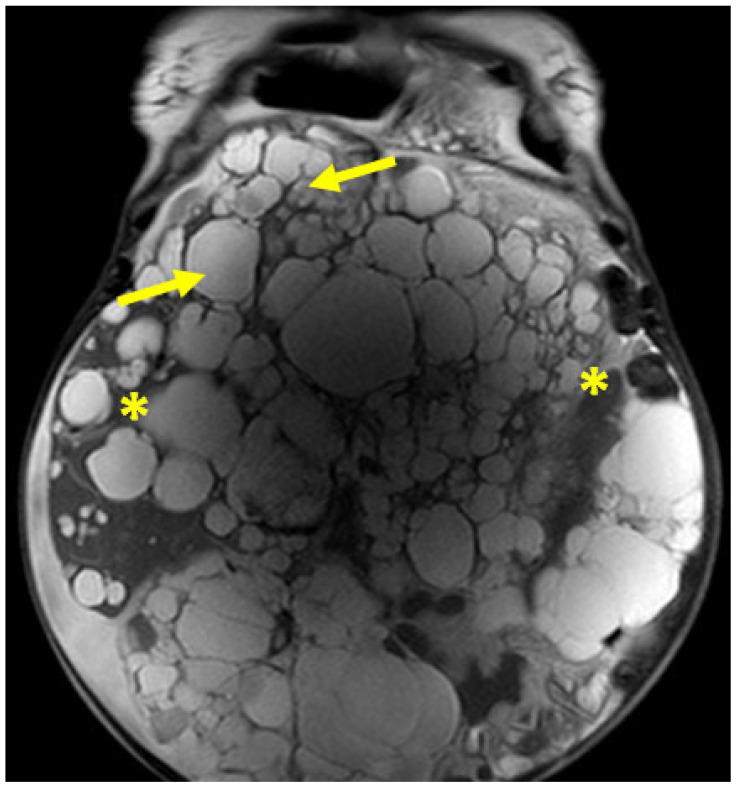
ADPKD body phenotype. Representative MRI scan demonstrating marked organomegaly in ADPKD. Axial MRI image showing bilateral massively enlarged polycystic kidneys (asterisks) and multiple hepatic cysts (arrows), characteristic of advanced ADPKD with severe organomegaly. Note the displacement of adjacent structures and significant reduction in available abdominal cavity space for other organs.
